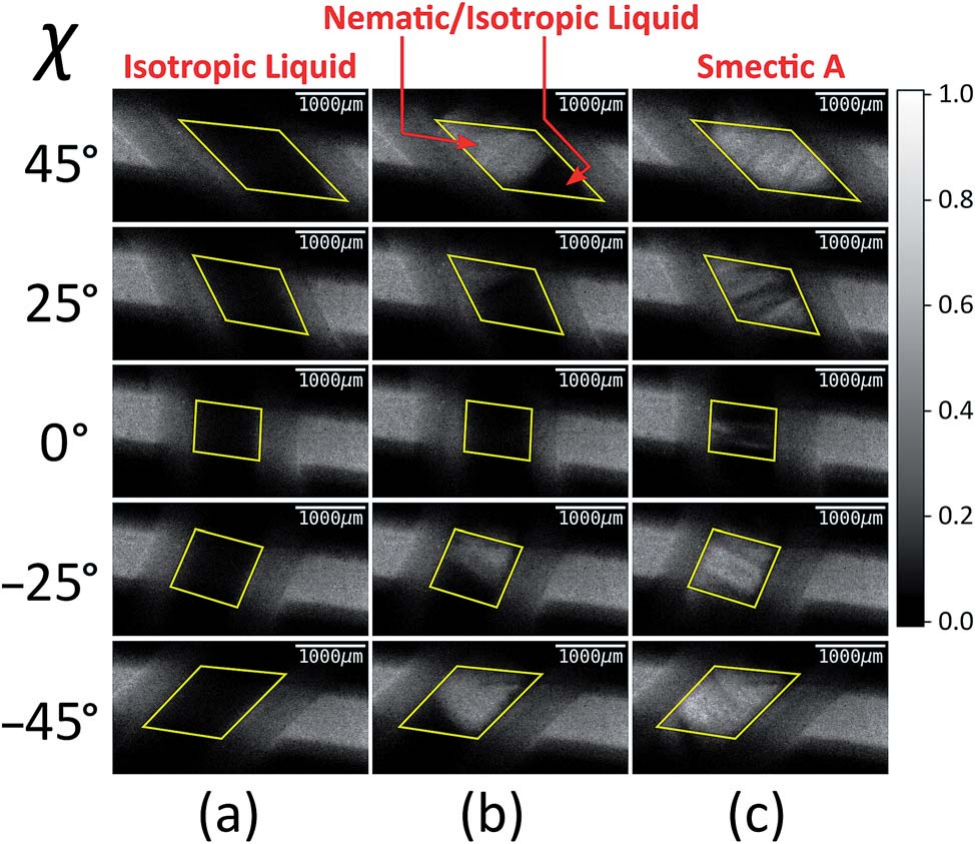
Selected XBI images recorded as a function of the orientation of the applied magnetic field (defined by angle *χ*) at the following temperatures: (a) 220 °C (isotropic liquid), (b) 214 °C (both nematic and isotropic liquid phases are present on account of a temperature gradient), and (c) 184 °C (smectic A phase). The scale of normalized X-ray intensity is shown on the right-hand side. The region of each XBI image representing the sample is defined by the yellow parallelogram.
